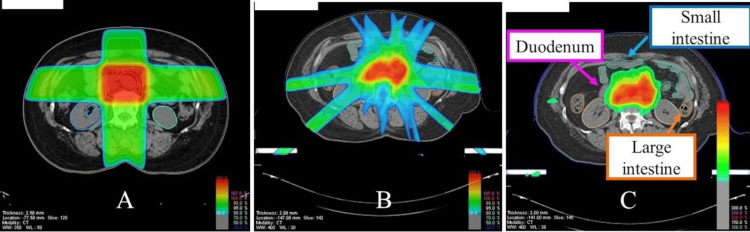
Typical scheme of re-irradiation (re-RT) planning (A) Previous radiotherapy planning record superimposed on the re-irradiation planning computed tomography (CT). (B) Re-irradiation radiotherapy record superimposed on the re-irradiation planning CT. (C) Cumulative dose displayed on the most recent planning CT. The organs at risk (duodenum, small and large intestines) were also delineated using that CT.
